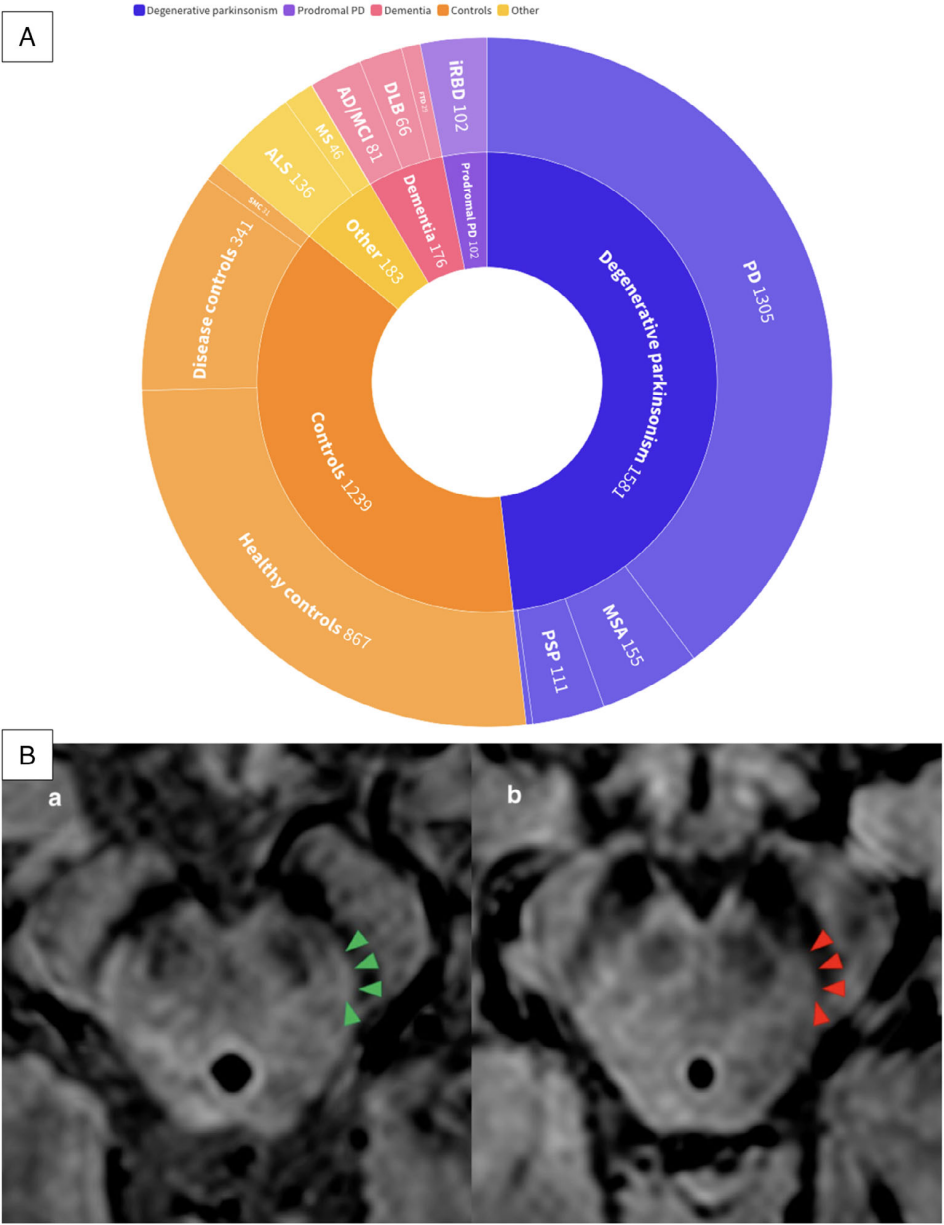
(A) Sunburst chart of the eligible studies’ population (interactive version: https://public.flourish.studio/visualisation/15954619/). (B) Swallow tail sign (STS) evaluation in high‐resolution MRI. STS presence (a) and absence (b). Reproduced with permission from Michler et al.^31^
